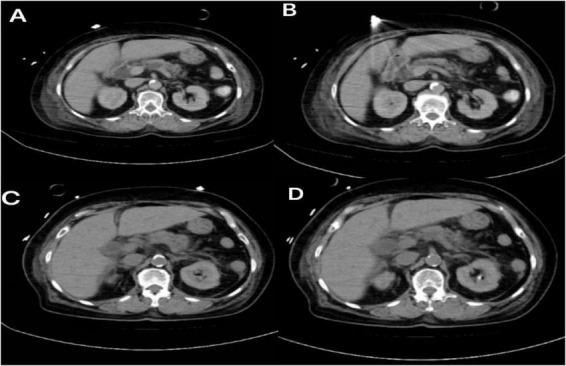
Representative non-contrast abdominal computed tomography images (A–D) of Case 1 demonstrate diffuse pancreatic swelling, along with edematous change in the duodenal wall near the bulb.
